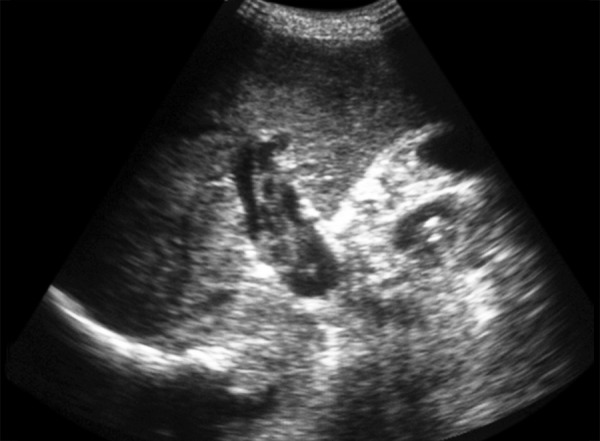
Ultrasonography showed dilated and obstructed portal vein
